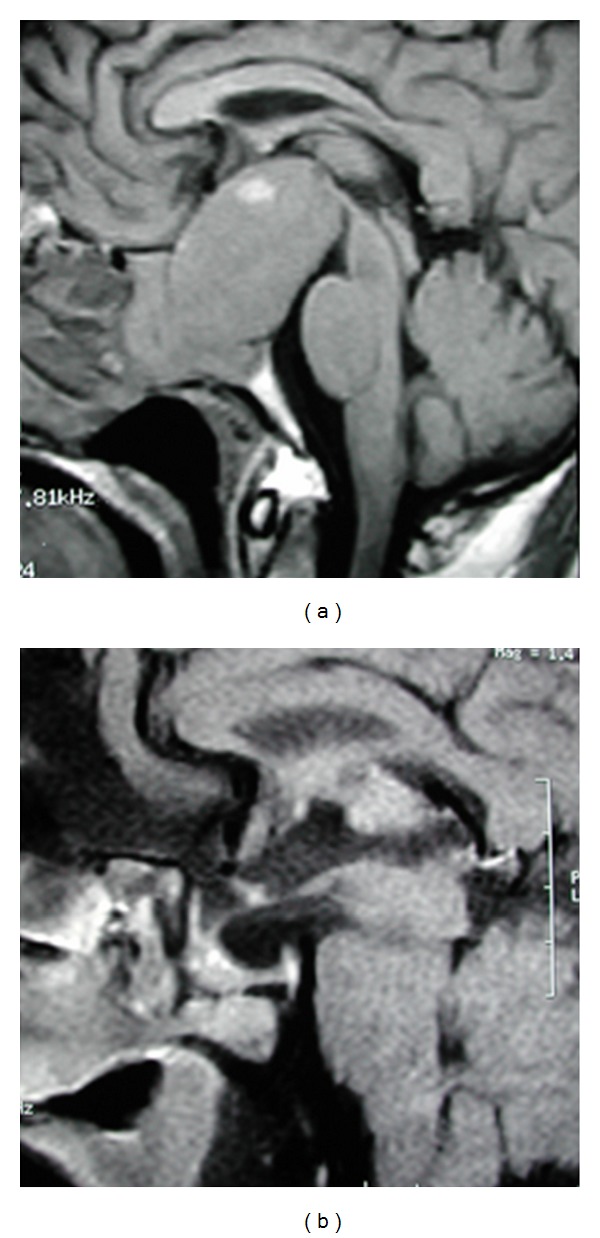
Enhanced MRI sagittal view that shows a giant pituitary adenoma reaching the third ventricle. Considering the uniform shape of the tumor, it could be removed through TS approach. (a) Preoperative view and (b) postoperative result.
